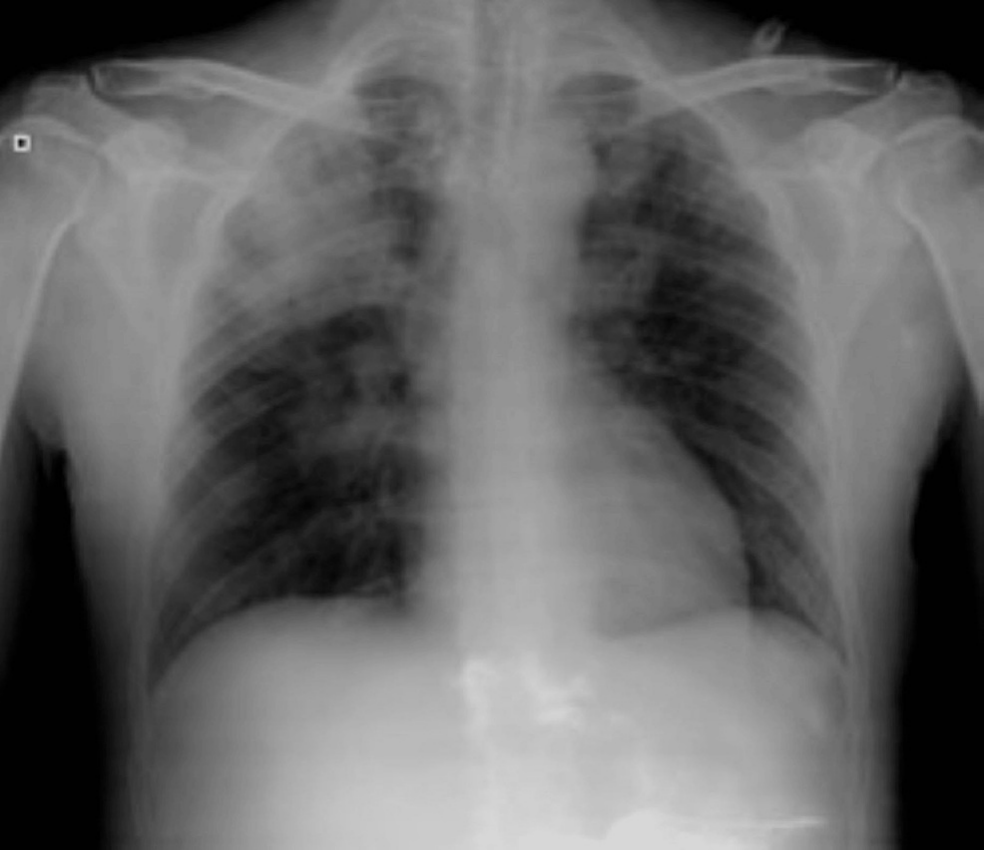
Chest X-ray Bilateral infiltrates in the upper pulmonary fields
